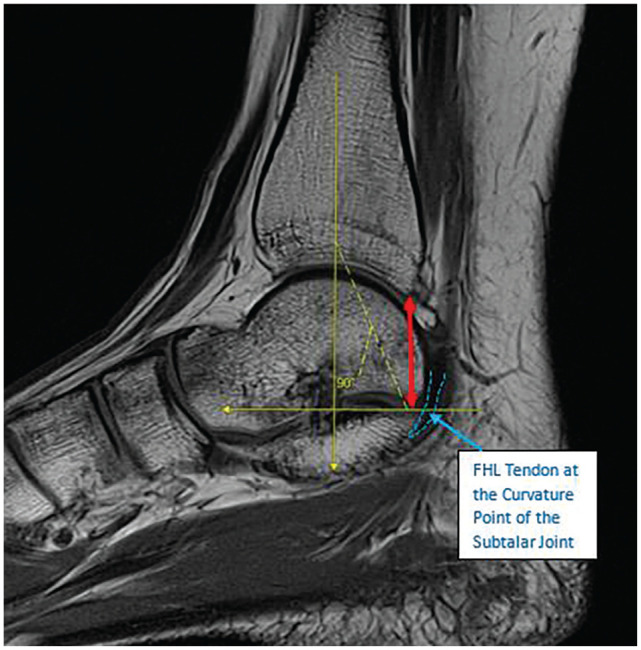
Mid-sagittal PD-weighted MRI shows a 22.5-mm tibia to tarsal tunnel measurement (red arrow) on the right ankle of a 15-year-old female with OT syndrome. The distance from the posteroinferior corner of the distal tibia to the curvature point at the subtalar joint (horizontal yellow line) is measured parallel to the tibial shaft (vertical yellow line). FHL, flexor hallucis longus; MRI, magnetic resonance imaging; OT, os trigonum; PD, proton density.
